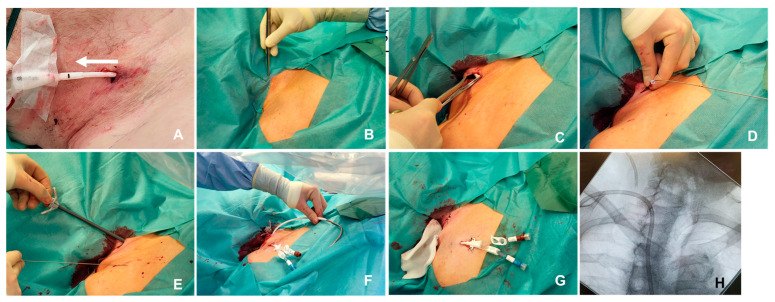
Step-by-step pictures of the modified procedure (A): The temporary CVC is pulled back slightly and fixated. (B): The old CVC is completely clear of the sterile field; the insertion is denoted by forceps. (C): Through a separate incision, the old catheter is dissected. (D): A guidewire is inserted and the old catheter is removed. (E): Insertion of a peel-away sheath. (F): Tunneling of the TCVC. (G) Completed procedure. (H): X-ray control with both the old and new catheters.
